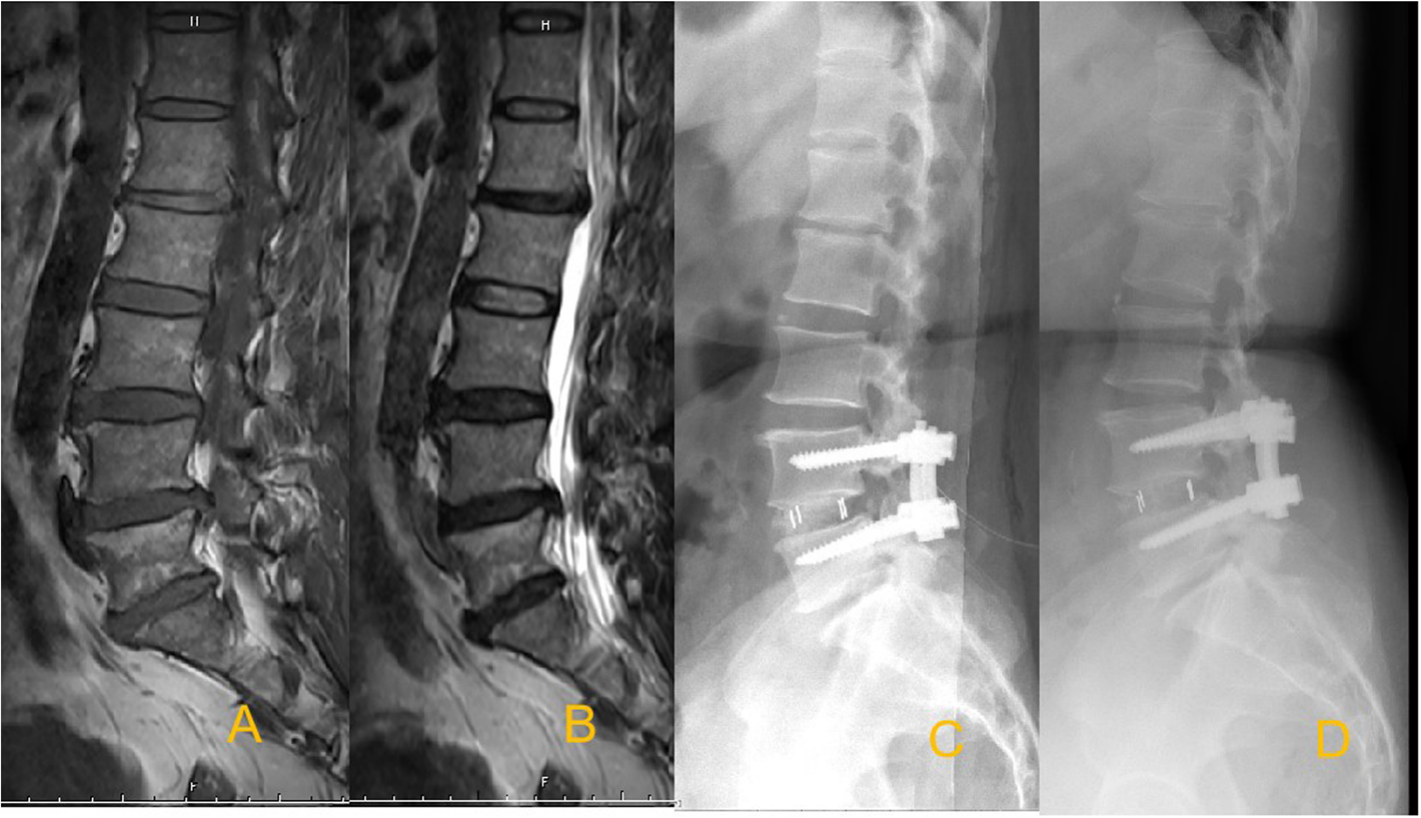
Sagittal T1-weighted (A) and T2-weighted (B) magnetic resonance images demonstrate L4−L5 lumbar disc herniation with MCs-2 signs. Postoperative radiographs (C) at the most recent follow-up confirming the solid fusion (D)
